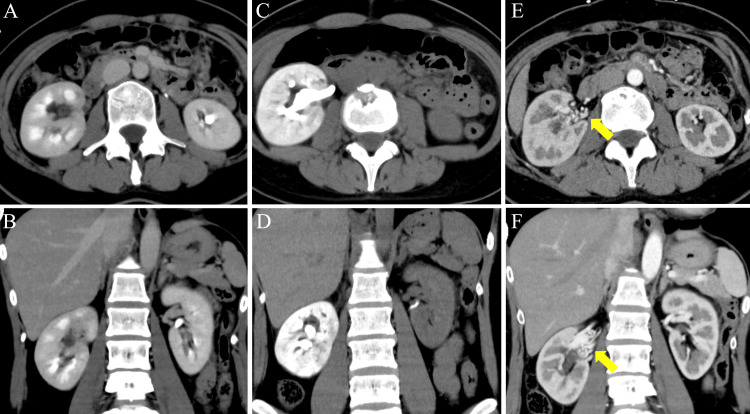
Computed tomography (A) Axial image. (B) Coronal image. The initial contrast-enhanced computed tomography (CT) in the equilibrium phase revealed delayed contrast excretion in the right kidney. (C) Axial image. (D) Coronal image. A subsequent plain CT demonstrated contrast pooling in the right kidney along with hydronephrosis. (E) Axial image. (F) Coronal image. The initial contrast-enhanced CT revealed small vessels in the right renal hilum, indicative of potential vascular malformations (yellow arrow).
